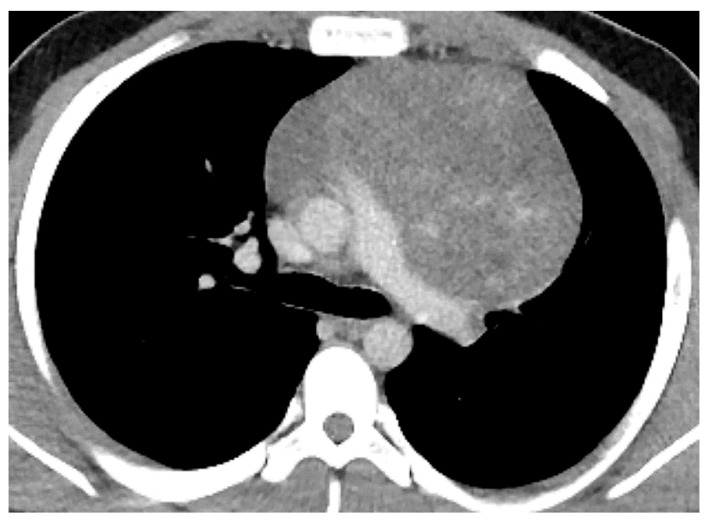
Nonseminomatous germ cell tumor (NSGCT). CT shows a large heterogeneous mass in the prevascular mediastinum. Biopsy showed teratoma with malignant transformation.
